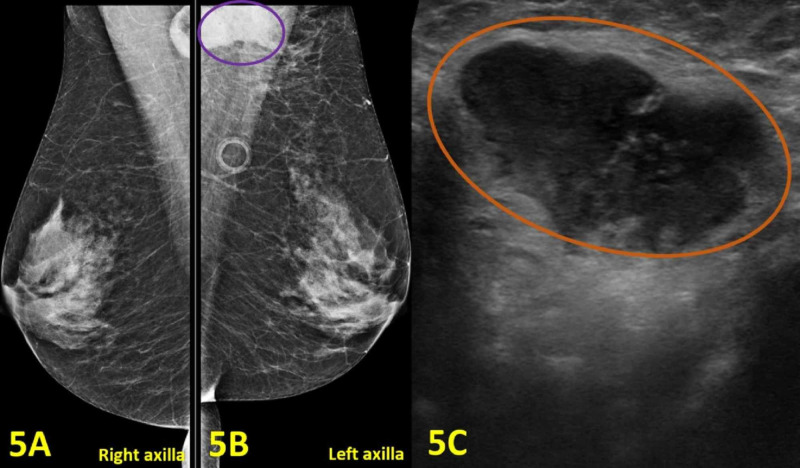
Imaging of Bilateral Breasts, Bilateral Axillae, and Left Axillary Lymphadenopathy (A) Mammogram of the right breast and axilla shows no abnormal findings. (B) Diagnostic mammogram of the left breast and axilla shows an enlarged lymph node with adjacent abscess measured 30 x 17 mm (purple oval). (C) Left axilla diagnostic ultrasound demonstrates an enlarged axillary lymph node with cortical thickening measuring 30 mm (orange oval).
